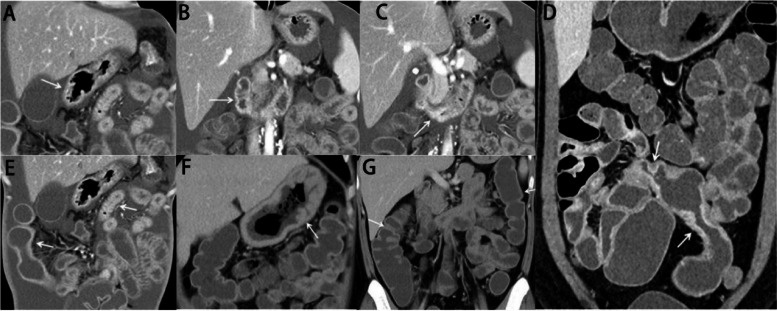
Representative CTE images of CEAS patients. A-C, computed tomography enteroscopy (CTE) images of patient 1, showing wall thickening of gastric antrum (arrow in A), descending duodenum (arrow in B) and horizontal duodenum (arrow in C) with enhanced mucosa. D, the CTE image of patient 11, showing thickened intestinal wall of multi-segmental ileum, enhanced mucosa, short-segmental stenosis (indicated by white arrows), and dilated intestinal lumen between strictured segments. E, the CTE image of patient 1 showing diffuse wall thickening of ileum with enhanced mucosa and multiple stenosis of ileum highlighted by white arrows. F and G, CTE images of patient 3, showing diffusely thickened gastric wall, thickened plicae and mildly enhanced gastric wall (arrow in F), and slightly thickened colon wall as well (arrows in G)
